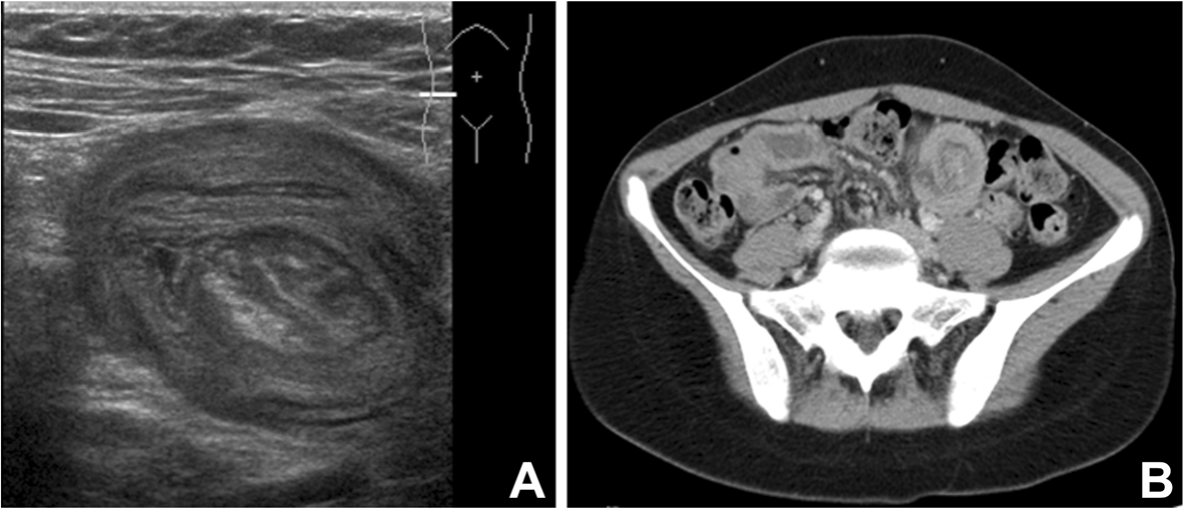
Radiographic evidences of the suspected intussusception. (A) Initial typical ultrasound demonstration of intussusception. (B) Computed tomography scan evidence of intussusception recurrence at day 5 after laparoscopic exploration.
